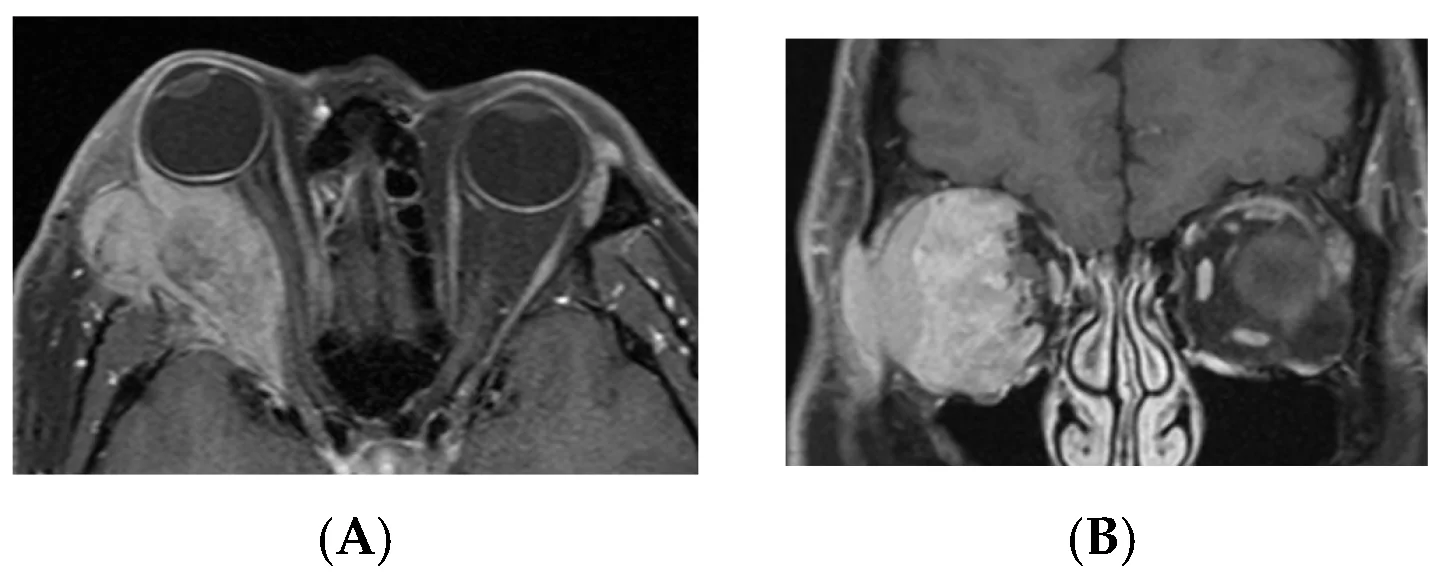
A 41-year-old man presented with about 6–8 months history of progressive proptosis and pain in the right orbital area. An incisional biopsy prior to referral confirmed the diagnosis of adenoid cystic carcinoma. (A,B) MRI findings, axial (A) and coronal (B) T1 weighted post-contrast fat-suppressed images show a hypointense homogeneously enhancing mass centered at the right lateral orbit measuring approximately 4.2 × 5.9 × 4.3 cm. Laterally, this mass invades the lateral orbital wall with invasion of the anterior aspect of the temporalis muscle. There is also involvement of the greater sphenoid wing, frontal process of the sphenoid, orbital floor, and orbital roof. Posteriorly, the mass extends to the orbital apex with involvement of the infraorbital foramen. There is infiltration of all extraocular muscles except for the medial rectus muscle. This patient had an orbital exenteration including resection of the sphenoid bone and extra-orbital component of the lesion followed by a free flap reconstruction and post-operative adjuvant radiation therapy.
